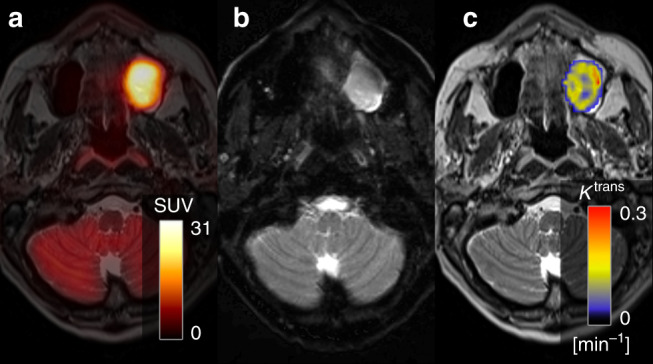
Example of multiparametric imaging with FDG uptake, diffusion and perfusion of a patient with a T2N0M0 tumour in the right maxilla involving both the maxillary sinus and the oral cavity. a depicts the fused FDG-PET/MRI scan, b depicts the diffusion-weighted imaging and c the dynamic contrast enhancement.
